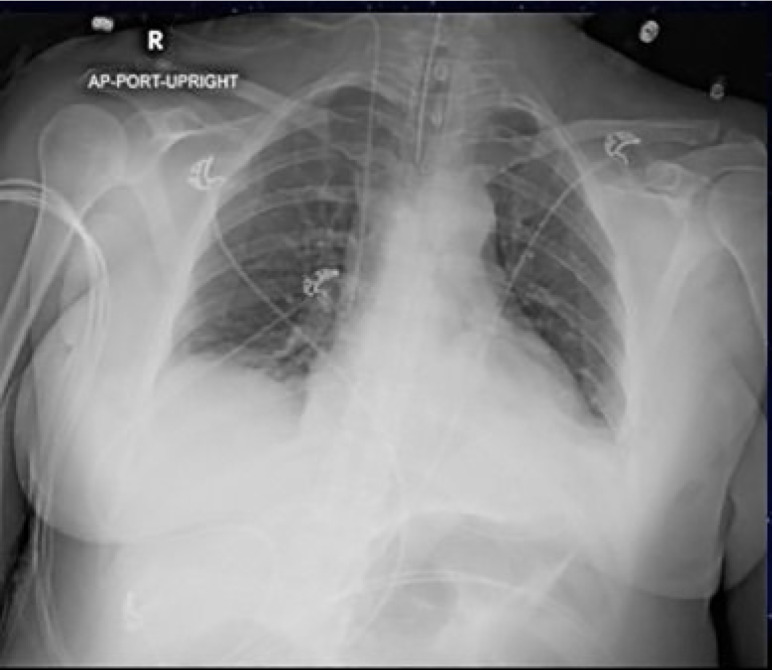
Chest radiograph of a 40-year-old female with sudden onset dyspnea.
